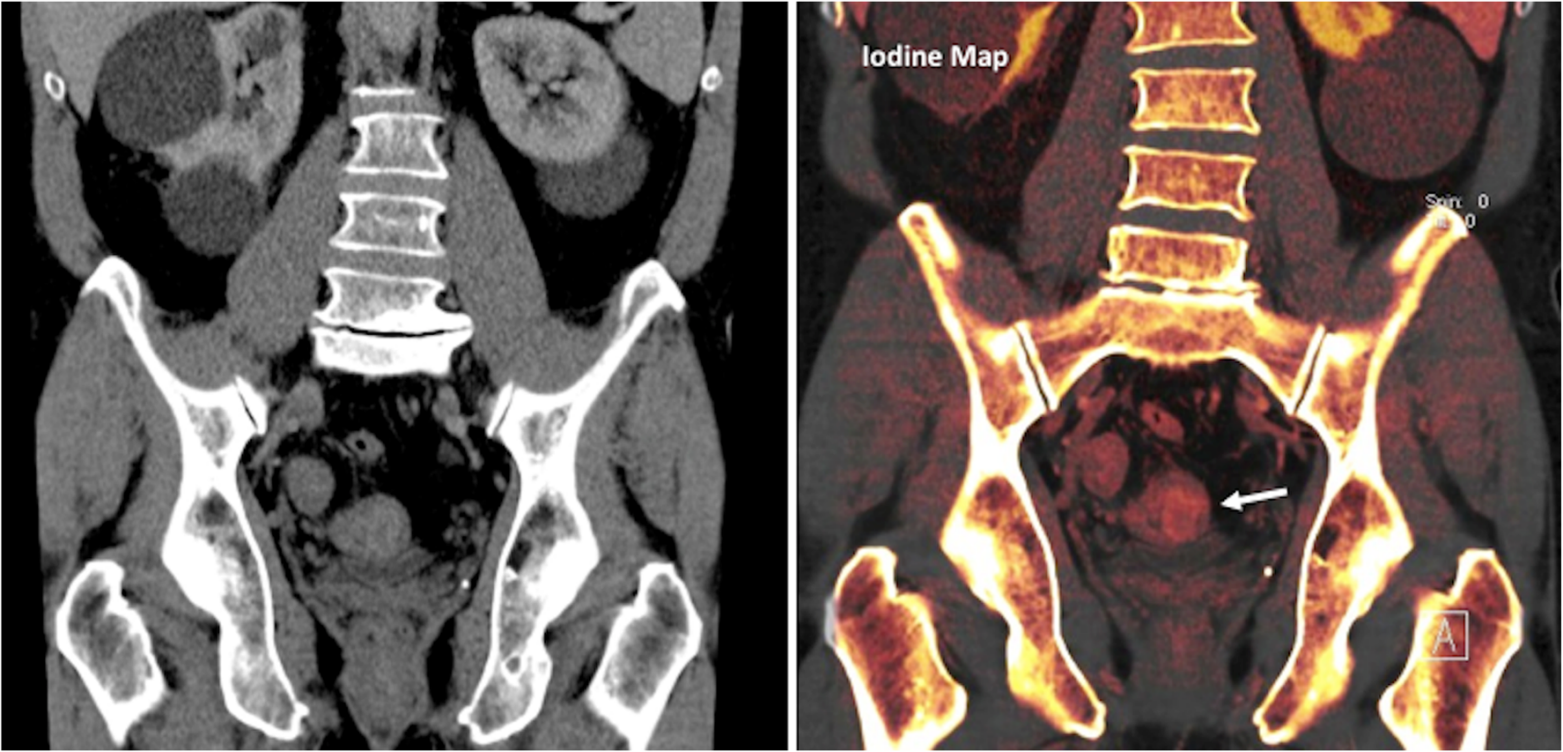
Dual-energy CT in a patient with a rectosigmoid tumour. Coronal reformatted conventional CT (left) and iodine map images. Iodine map depicted iodine uptake in a mass in the sigmoid colon (arrow), improving tumour detection on CT exams without bowel preparation
